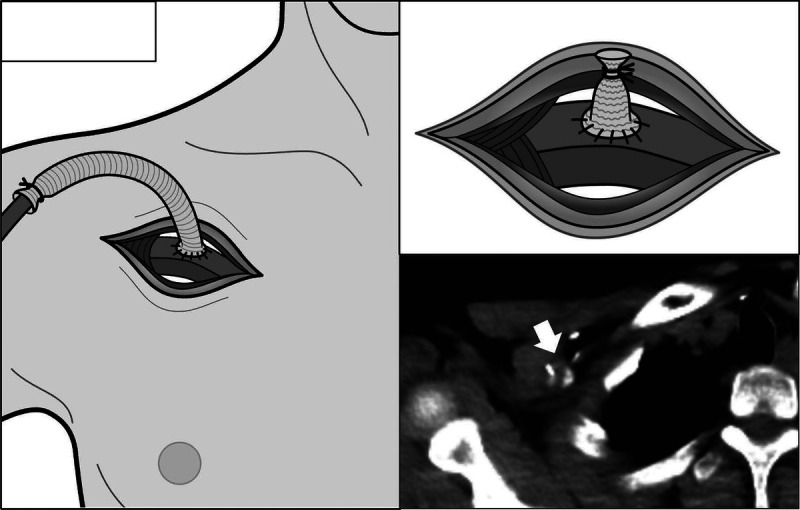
A prosthesis is sewn into insertion sites of the extracorporeal-circulation device, in the axillary or femoral artery (left); when the device is weaned, the root of the graft is ligated and the device side is excised (right). Mild-to-moderate FDG uptake has been reported even in noninfected vascular grafts.^11^ The present case was not confirmed pathologically, but was diagnosed as nonspecific FDG uptake in areas of scarring and prostheses consistent with the device insertion route more than 10 years ago. To properly diagnose malignant disease on FDG PET/CT, including PTLD after cardiac transplantation, it is necessary to know that FDG uptake may occur at the site of insertion of any previously inserted device.
